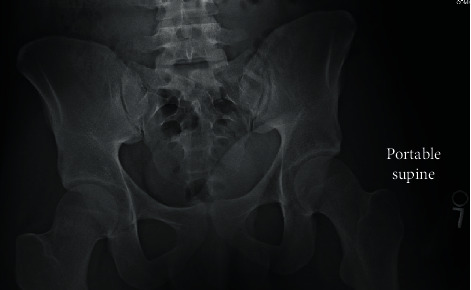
6-month postoperative anteroposterior X-ray of the pelvis. This radiograph demonstrates complete excision of the exophytic mass from the patient's left iliac wing without any sign of recurrence (Osteochondroma 6 month post-op follow up.jpg).
